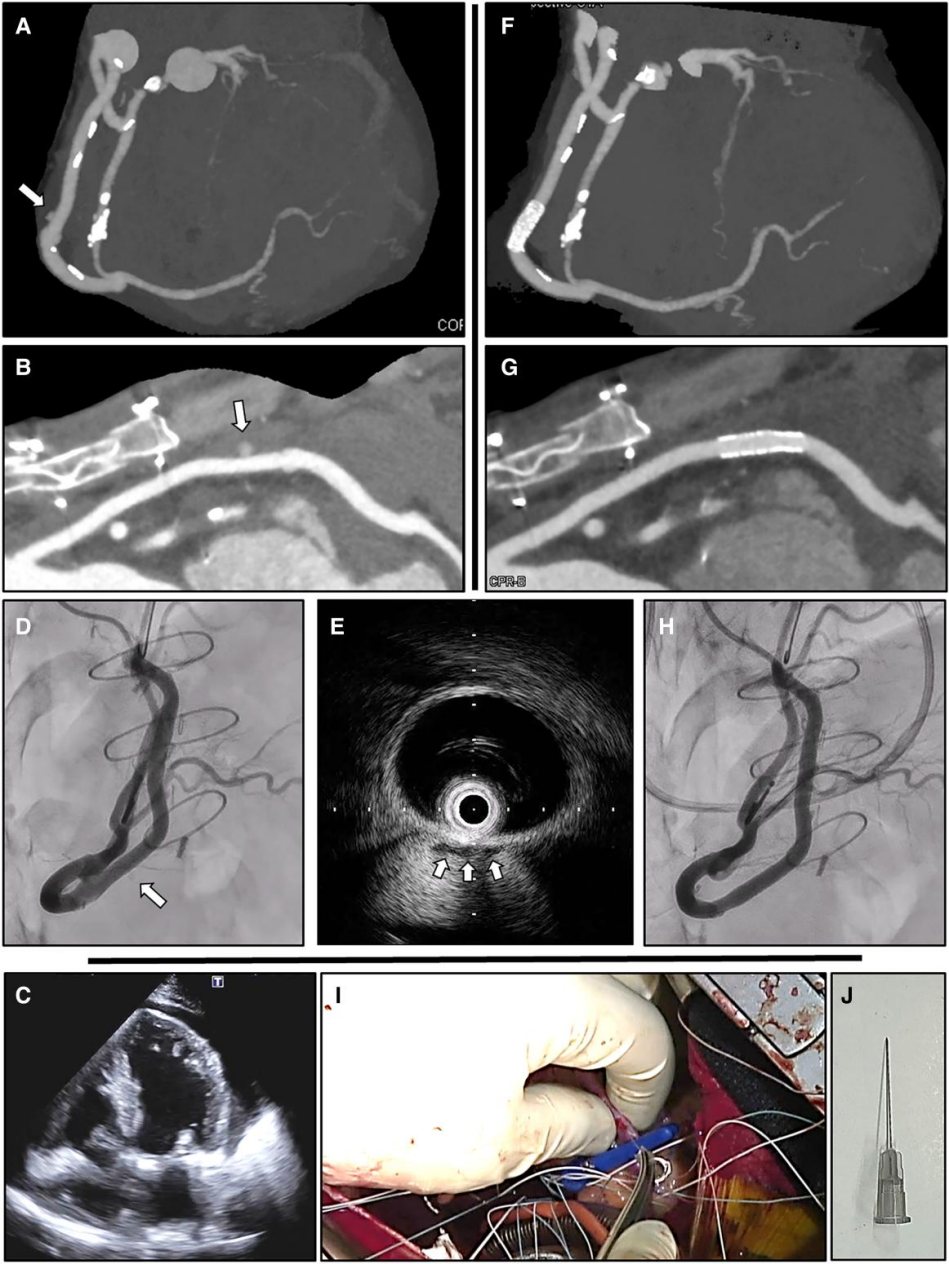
Successful haemostasis for bleeding from a saphenous vein graft needle hole. A computed tomography scan showed contrast extravasation from the centre of the saphenous vein graft between the aorta and the distal right coronary artery (*A* and *B*, arrows). Persistent microbleeding resulted in cardiac tamponade (*C*). It was very difficult to determine the bleeding site on fluoroscopic images (*D*, arrow). After injecting contrast medium while observing intravascular ultrasound images and filling the vessel with negative echo contrast, the bleeding point was identified as the location where a small amount of negative echo contrast was present outside the vessel (*E*, arrows). Successful haemostasis was confirmed after implantation of a covered stent (*F–H*). The bleeding origin was thought to be a needle hole (27G) drilled to deaerate the grafted vein after coronary artery bypass surgery (*I* and *J*).
